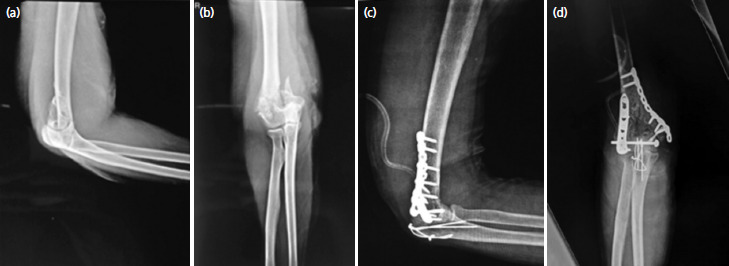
(a) Pre-operative radiograph of elbow after chevron’s osteotomy in AP and (b) lateral view of a 55-year-old male, a post-traumatic case of right sided distal humerus intra-articular fracture closed with no distal neurovascular deficit; Post-operative radiograph of elbow in (c) AP and (d) lateral view of the same patient.
